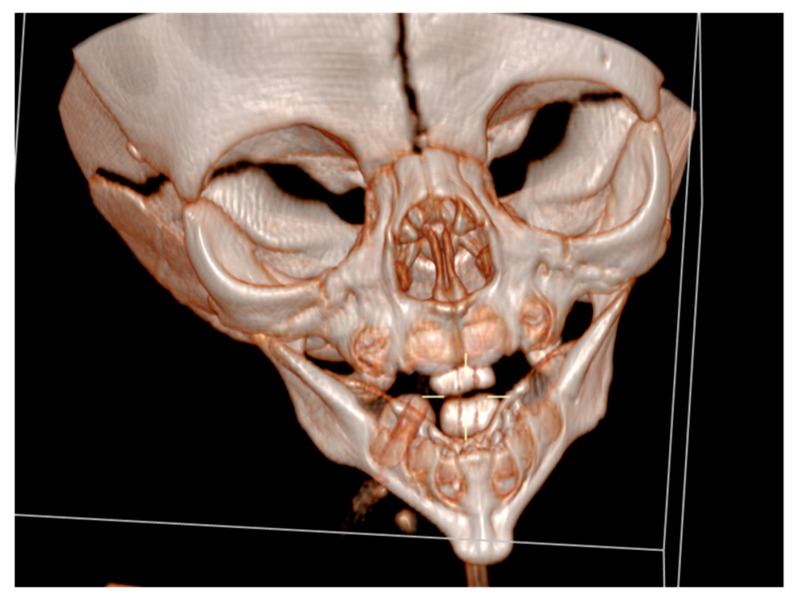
A computed tomography of a narrow and hypoplastic mandibula.
